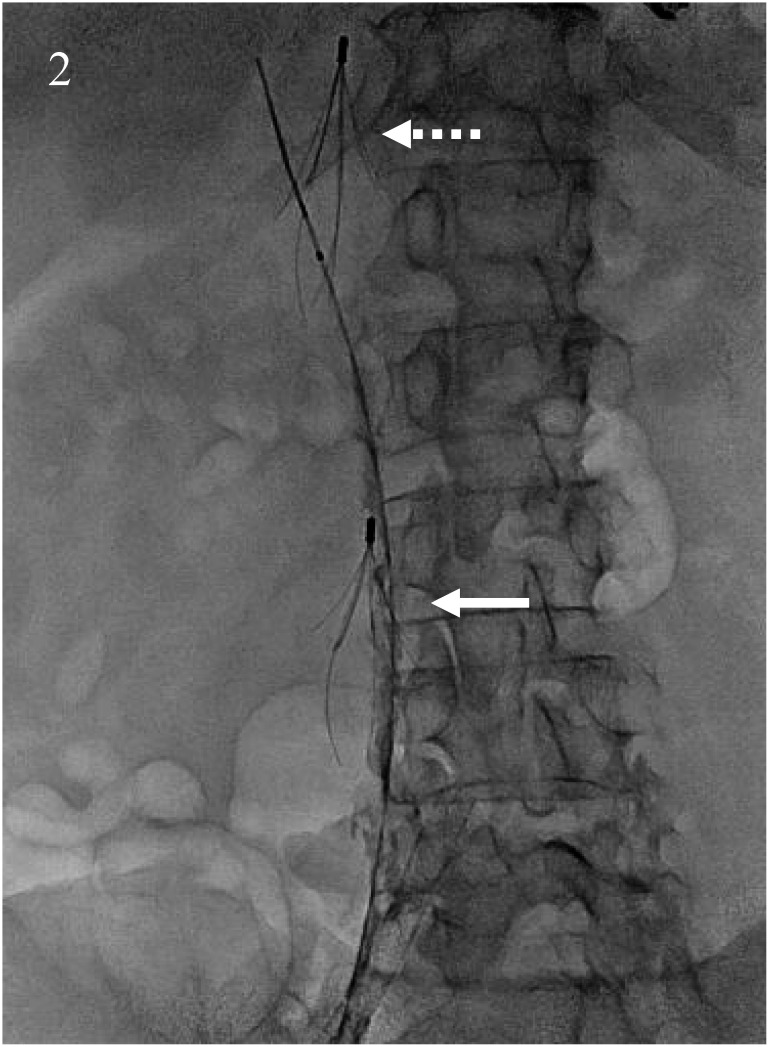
Fig. 2 Posteroanterior radiograph demonstrating the distal (white arrow), proximal (dashed arrow) inferior vena cava filters, and catheter-directed thrombosis catheter.
